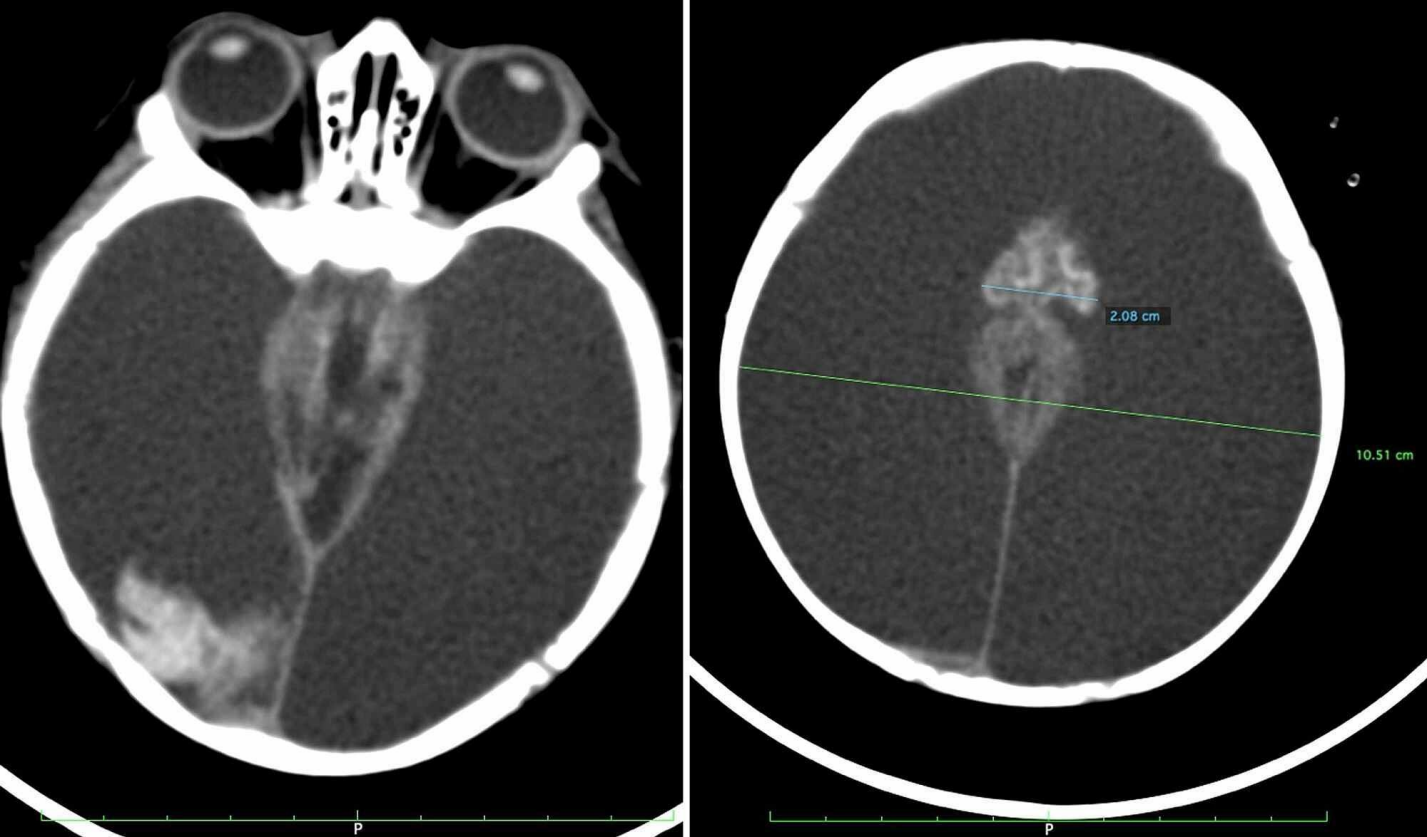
Massive subdural collection on CT scan one week after head trauma CT scan of the brain without contrast showing massive hypointense extracerebral fluid collection associated with hyperintense imaging, occupying the occipital region on the right side of the intracranial compartment (left). Brain collapse can seen at the midline (right).
